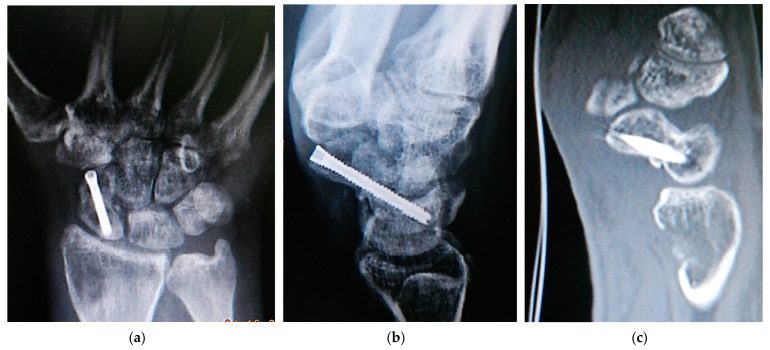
Postoperative radiologic investigation of scaphoid nonunion with resultant union achievement: (a) posteroanterior and (b) lateral x-rays showing scaphoid union and bone defect in the donor site of the distal Radius; (c) sagittal CT scan view showing incorporation of the pronator quadratus graft and the donor site bone defect.
